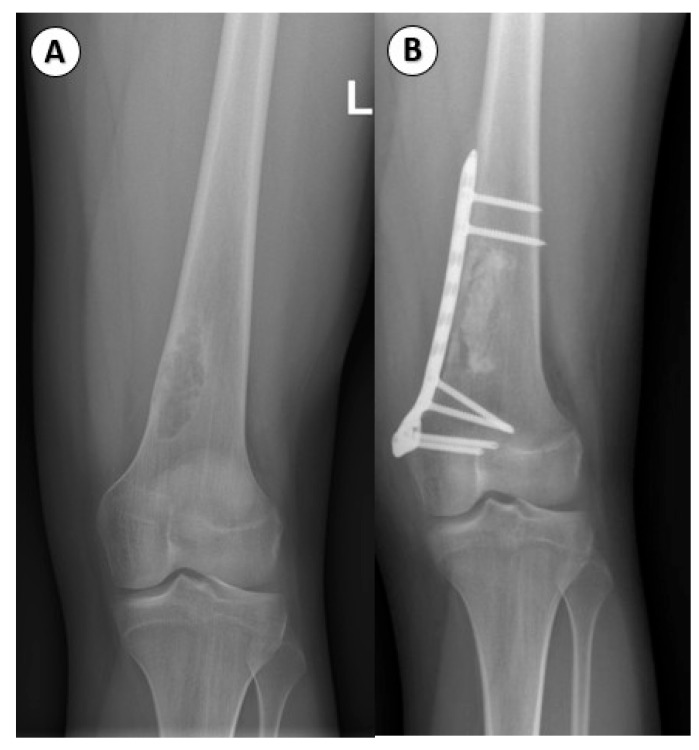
Osteoblastoma of the left femur. The radiograph shows a well-defined, expansile, lytic lesion in the metaphyseal region of the distal left femur, with patchy radiolucencies and peripheral rim of sclerotic bone (A). The patient later developed a pathological fracture. Treatment consisted of reduction and internal fixation. Postoperative appearance one year after surgical intervention (B).
